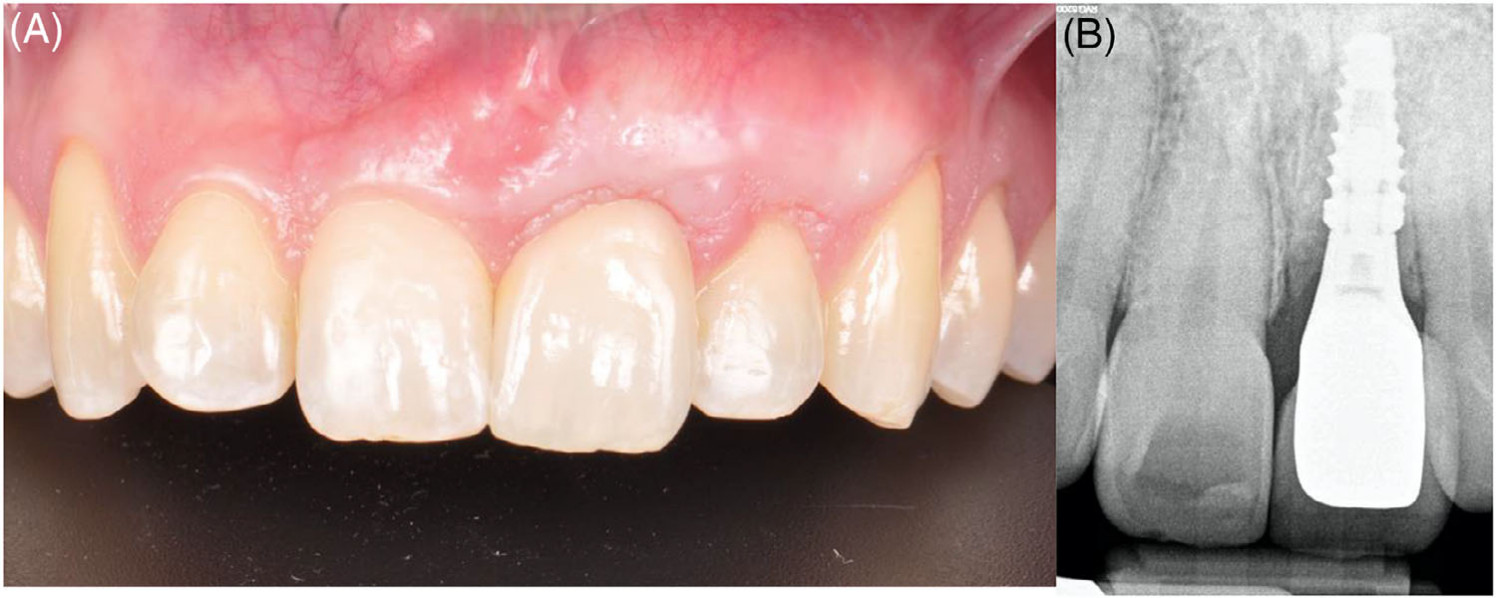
Final follow‐up. Frontal view (A). Periapical radiograph (B).
